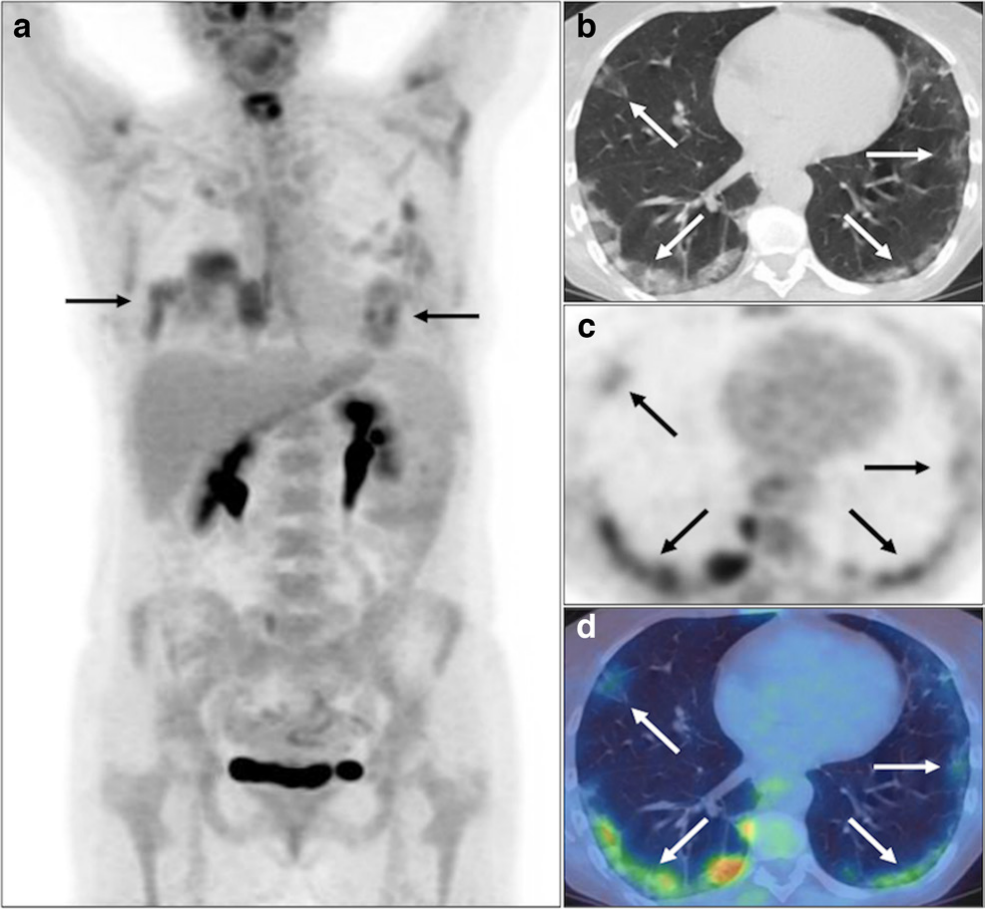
Bilateral FDG avid subpleural GGO and consolidation with perilobular opacity, *i.e.,* OP pattern (solid black and white arrows), and a mid-lower zone predominance, *e.g.,* right lower lobe (SUV_max_ 6.0) on PET MIP (A), axial CT, PET and fused PET-CT (B-D). BSTI 1 with positive RT-PCR (*confirmed* case).
